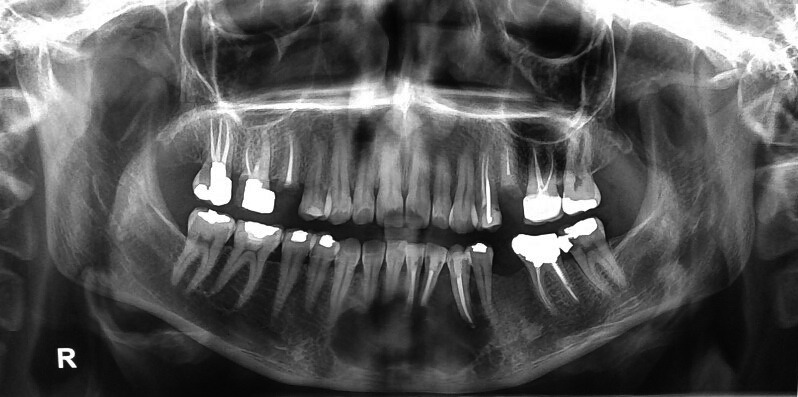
Initial orthopantomography (OPG) obtained from the patient upon presentation to our dental office. Notice the extension and multilocular pattern of radiolucency
